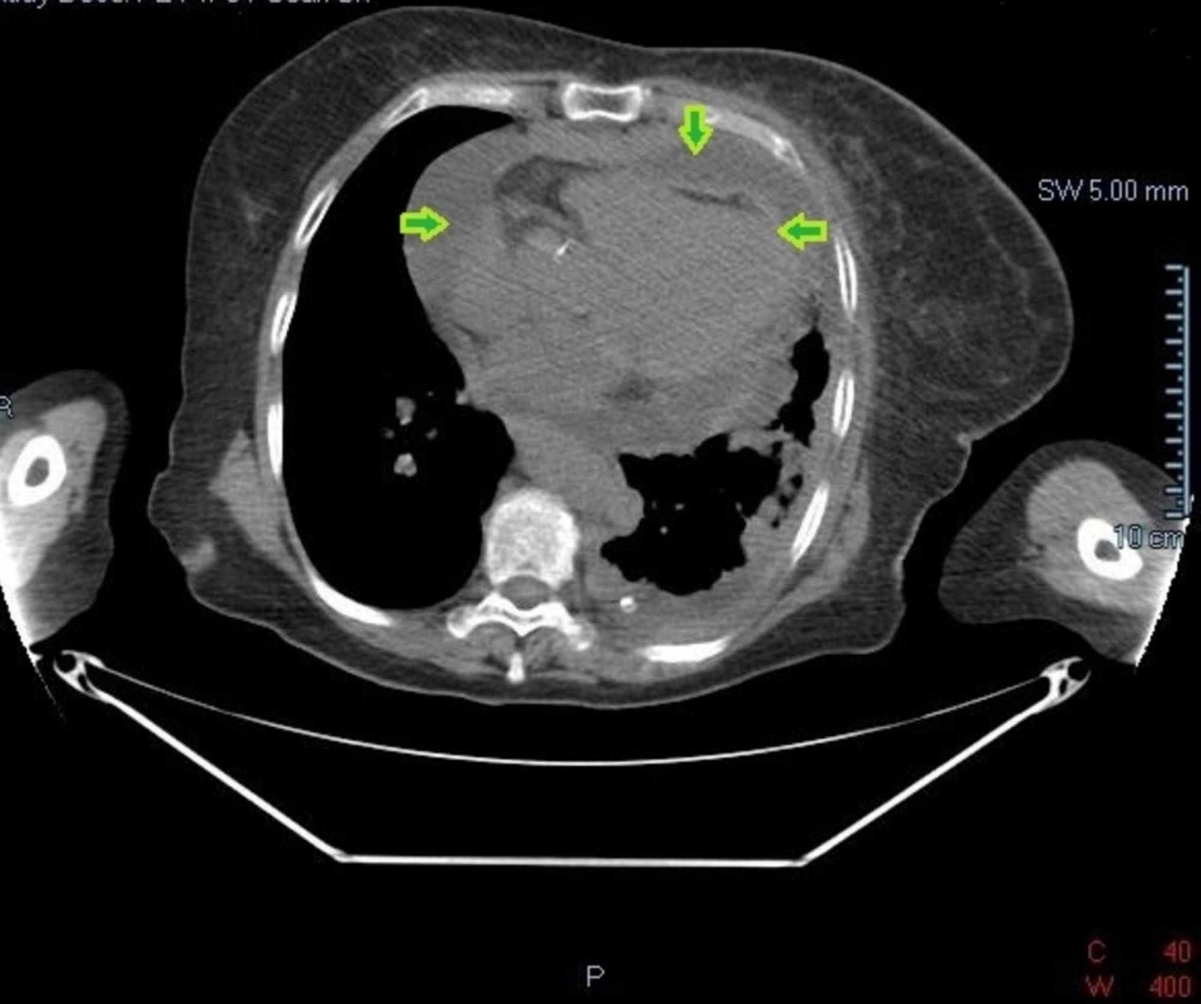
CT chest shows moderate sized pericardial effusion (green arrows).
